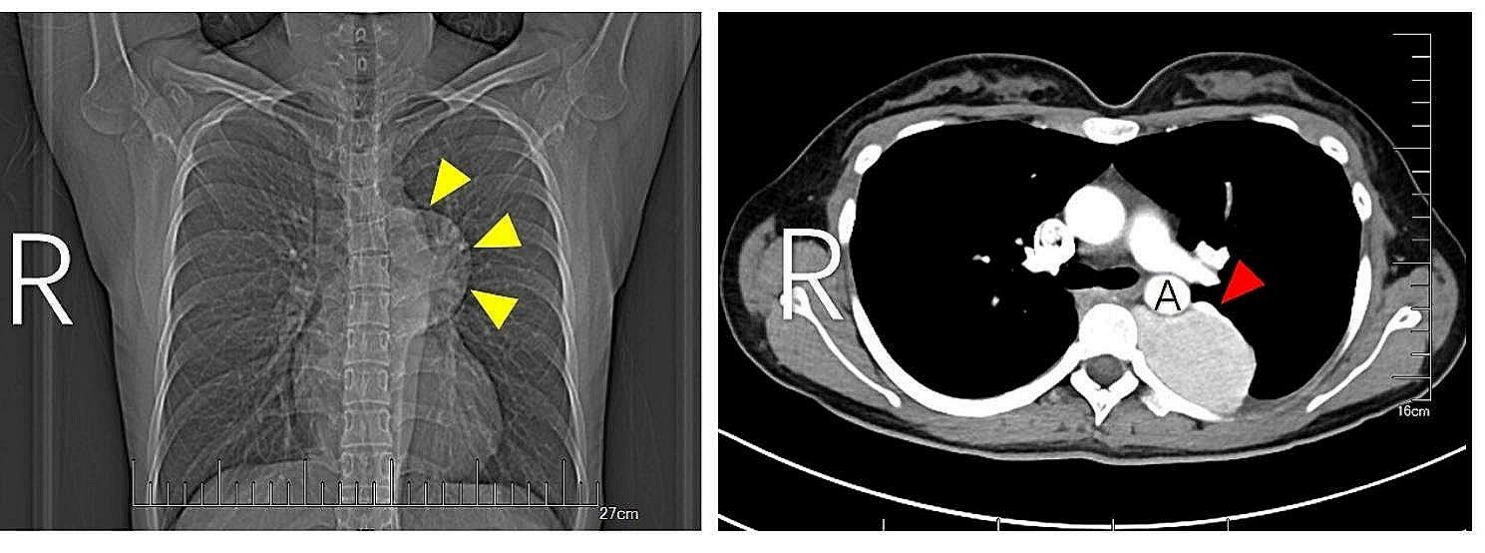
Chest radiography and contrast-enhanced computed tomography (CT) findings. Chest radiography demonstrated the presence of a mass within the left thoracic cavity, as indicated by yellow arrows. Contrast-enhanced CT scans exhibited a soft tissue density measuring approximately 39 mm×60 mm. A tortuous vein was observed encircling the surface of the mass, with drainage into the innominate vein. A, aorta
